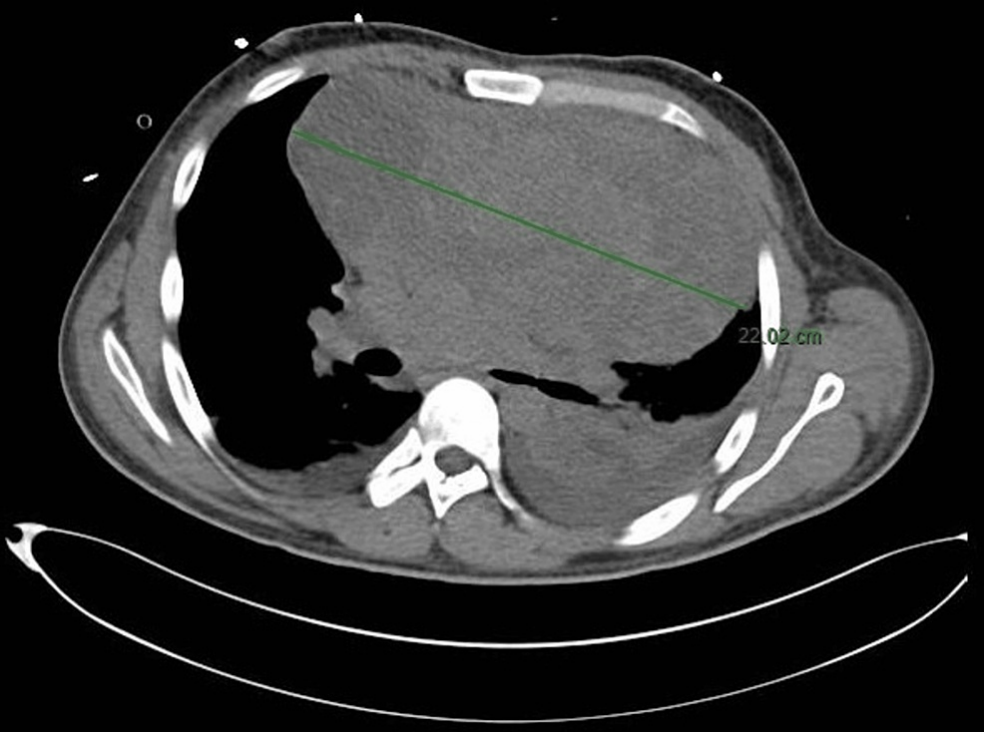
A CT scan of the thorax shows an anterior mediastinal mass of 22 cm in largest diameter. It is causing significant compression of the left lung.
